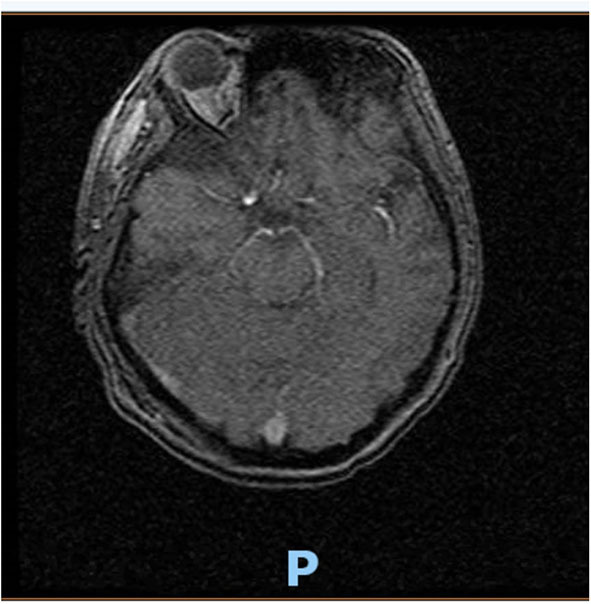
MRI brain (cross-section at pons) consistent with acute infarct of pons.
